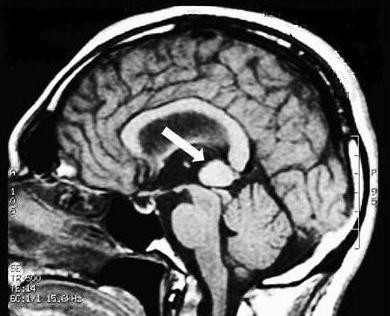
Magnetic Resonance Imaging (MRI). The MR scan of the brain using contrast medium (gadolinium) showed a large pineal cavernoma.
